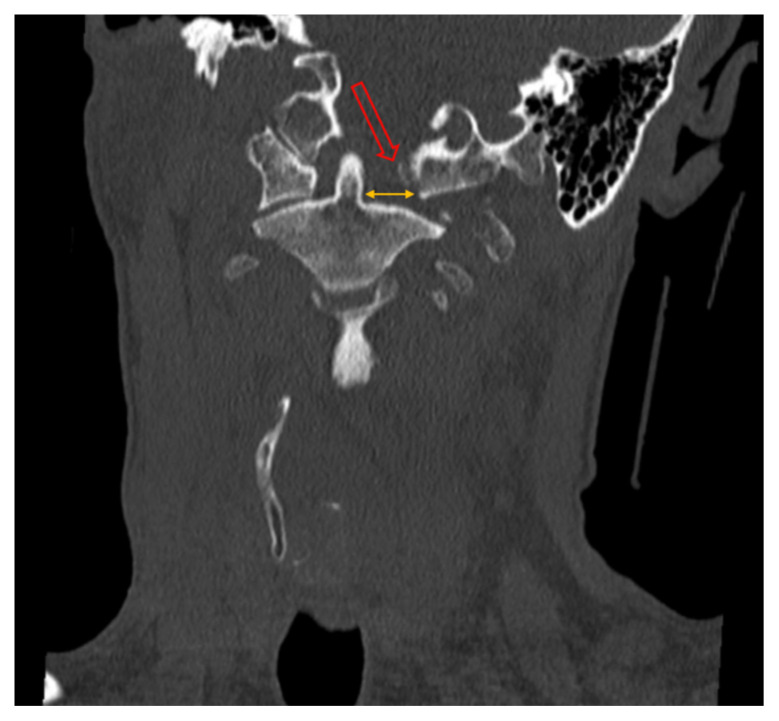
Coronal CT Video Link: https://youtu.be/f3C6Zor7Wes
